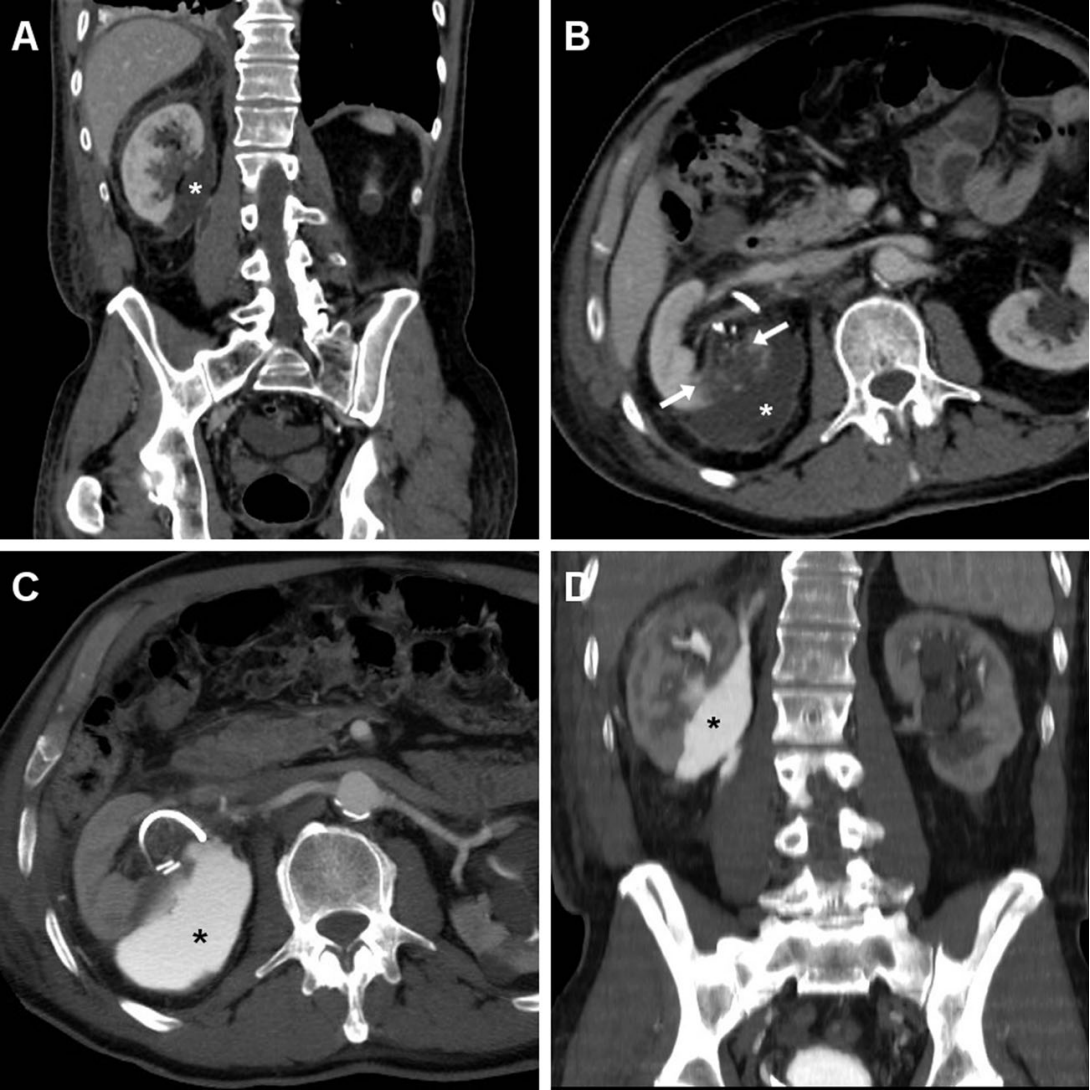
Peri-renal urinoma in a 73-year-old man investigated with multidetector CT after laparoscopic resection of right-sided renal tumour because of progressive blood loss and acute flank pain. Contrast-enhanced coronal (a) and axial (b) images showed a medial peri-renal fluid collection (*), ureteral stent in place and clips at the renal hilum, and a 2.5-cm hypoenhancing portion of the posterior renal cortex (*between arrows*) corresponding to the resection site. Forty-eight hours later, follow-up split-bolus CT-urography (c, d) showed minimally enlarged collection filled with enhanced urine consistent with urinoma from injured renal pelvis. Postoperative course included prolonged ureteral stenting and blood transfusions. (Partially reprinted with permission from Tonolini et al. [52])
